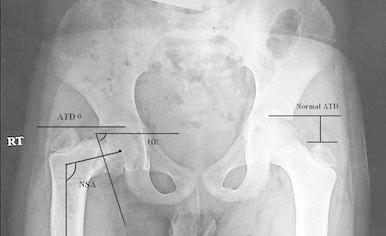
Radiographic evaluations on the standing anteroposterior radiographs with the hips in neutral position were performed. *a* Measurements of the Hilgenreiner’s epiphyseal angle (HE), neck-shaft angle (NSA), articulo-trochanteric distance (ATD)
